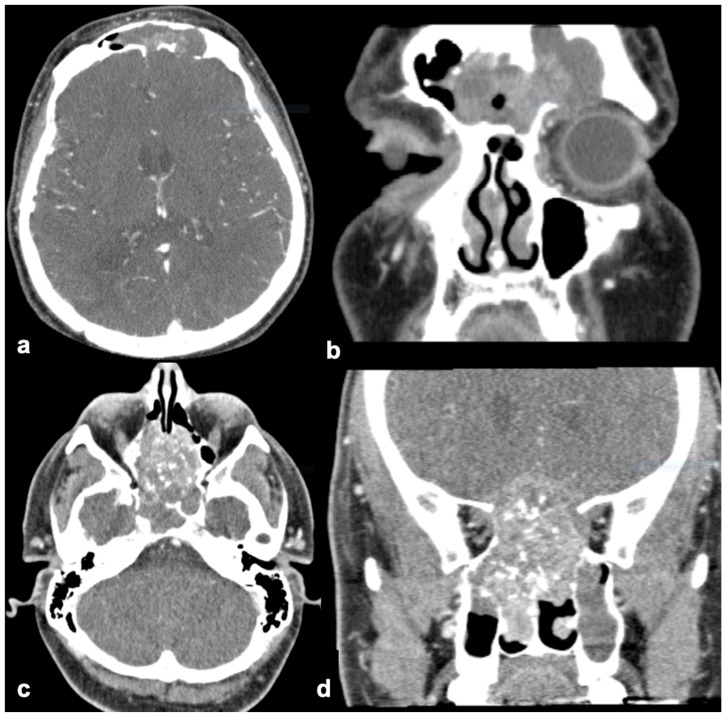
(a–d) Axial (left) and coronal (right) slices of two CT images of SNIP-SCC (case 1 (a,b) and case 2 (c,d)). Intracranial defects are observed in (a) posterior wall of frontal sinus and (b) skull base. Both tumors are bilateral (extending to both sides).
